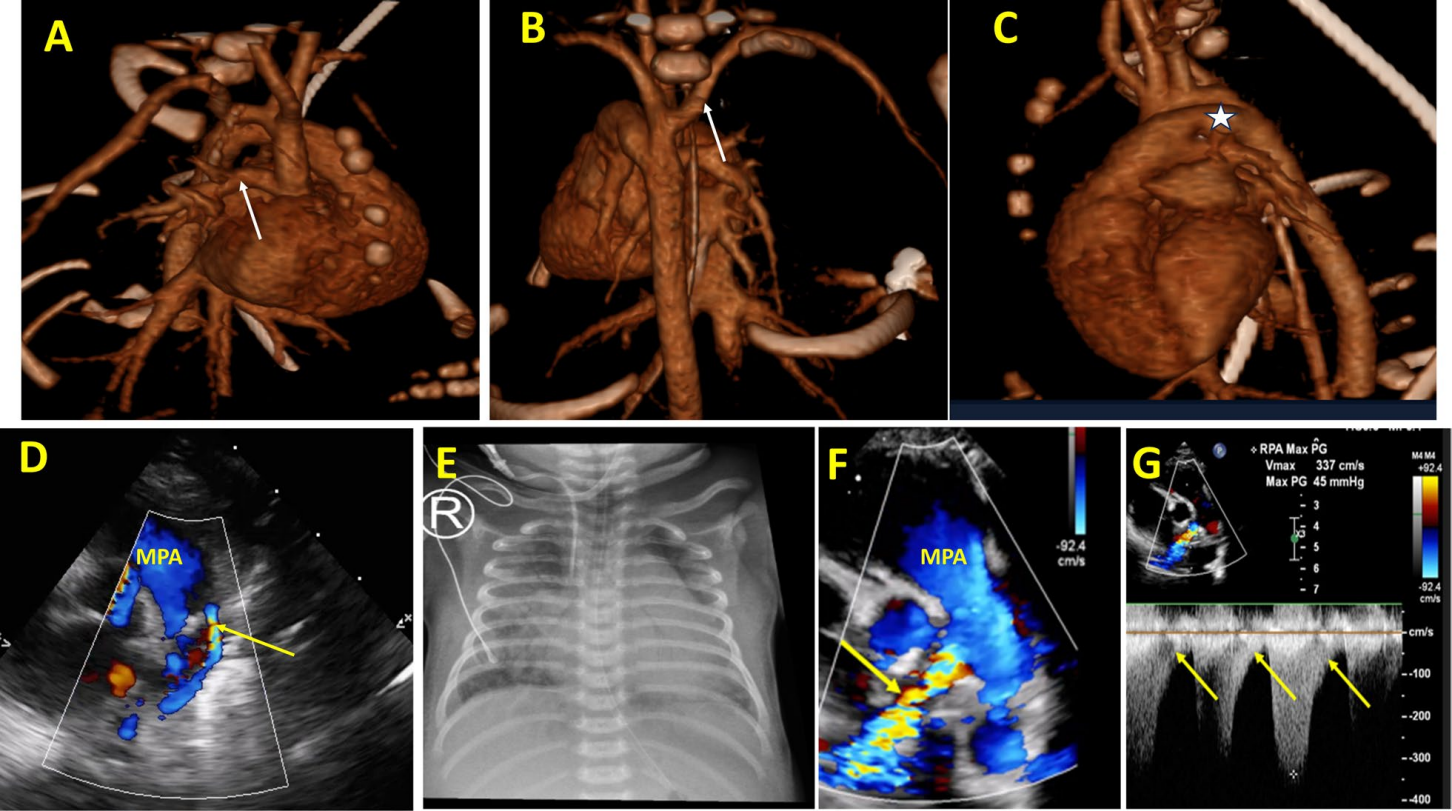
A MDCT angiography with 3D reconstruction and right lateral projection showing the anomalous RPA from the ascending aorta (white arrow). B MDCT angiography with 3D reconstruction and posterior projection showing the aberrant right subclavian artery (white arrow) with no coarctation of the aorta. C MDCT angiography with 3D reconstruction and left lateral projection showing the large PDA (white arrow) connected to the descending aorta. D The parasternal short-axis view shows constriction of the PDA with right-to-left shunting after the discontinuation of prostaglandins (yellow arrow). E Preoperative chest x-ray showing plethoric right lung. F The parasternal short-axis view shows postoperative RPA stenosis (yellow arrow). G continuous wave Doppler showing postoperative RPA stenosis with diastolic runoff (yellow arrows). MDCT: multidetector computed tomography, MPA: mean pulmonary artery, PDA: patent ductus arteriosus, RPA: right pulmonary artery
